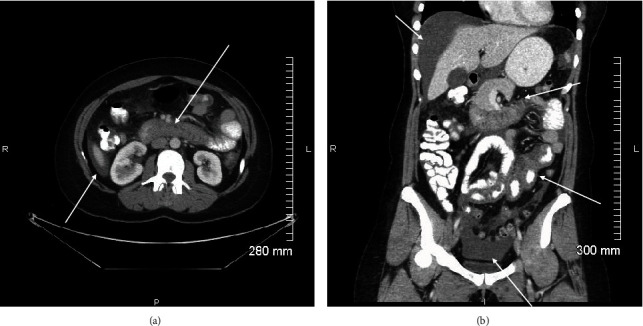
Axial (a) and coronal (b) contrast-enhanced CT scan of the abdomen and pelvis. Images demonstrate a moderate amount of abdominopelvic ascites with diffuse wall thickening, hyperenhancement, and mucosal edema of the entire small bowel.
